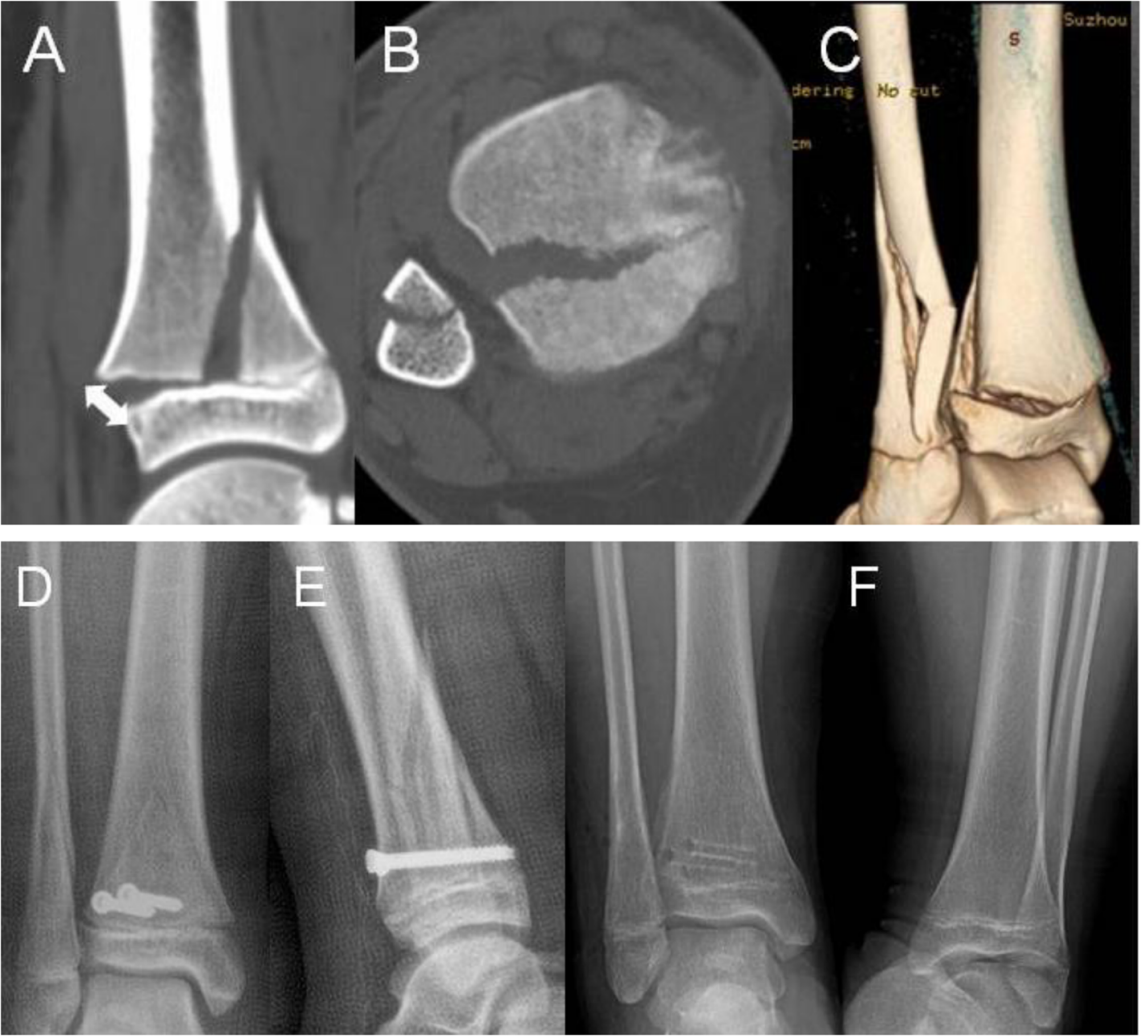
A 13-year-old boy. a–c, Sagittal, axial and 3D view demonstrated 8 mm displacement of the fracture (white double arrow). d, e AP and lateral radiographs demonstrated the anatomical reduction immediately post operatively. f AP radiograph of bilateral ankles obtained 7 months after ORIF showed PPC without any ankle deformity
